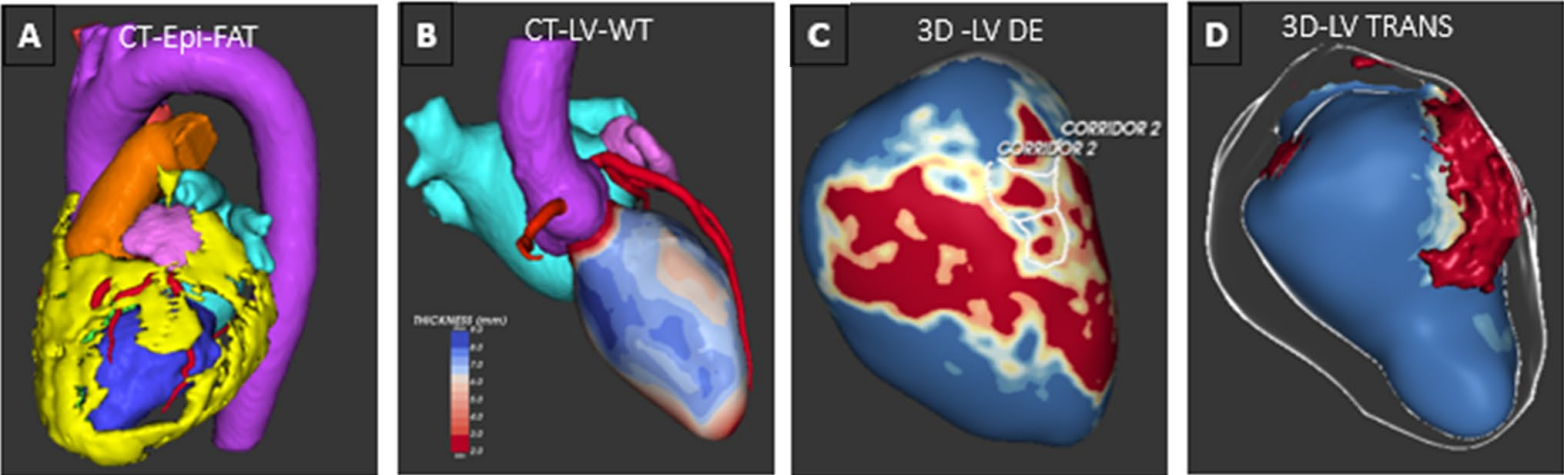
Structural substrate characterization: clinical imaging modalities to guide procedural planning (here, visualized in Adas3D; V2.11.0 2022, Galgo Medical, Spain). A Anatomical cardiac CT segmentation and extensive epicardial fat (yellow). B CT left ventricular wall thickness model combined with anatomical segmentation of aorta, coronaries and left atrium. C 3D CMR LGE scar model depicting scar core in red, border zone in white and healthy tissue in blue. Possible conductive channels are localized per myocardial layer and outlined by white lines. D Visualization of transmurality of scar in 3D CMR LGE model
